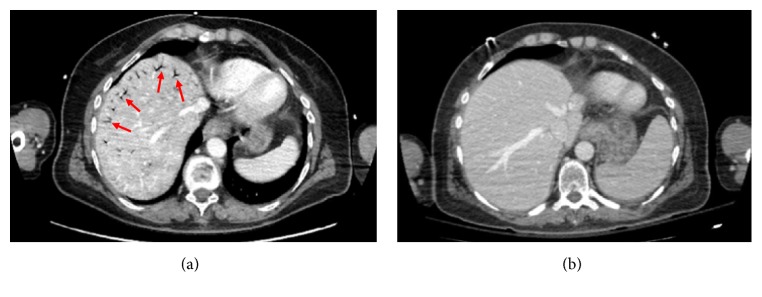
Abdominal CT scan: (a) GE in the peripheral branch of the portal system after HP ingestion; (b) reduction of the GE after HBOT.
